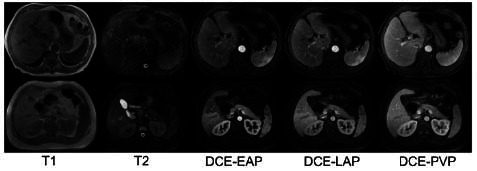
Different MRI sequences images.
